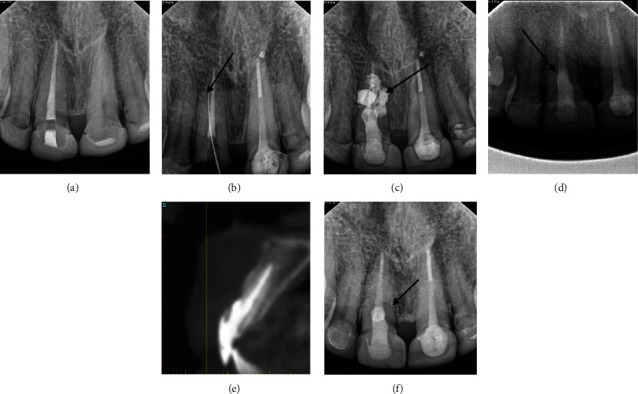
(a) Radiograph after root canal retreatment. (b) Radiograph showing root perforation after postspace preparation. (c) Calcium hydroxide placement. (d) MTA placement. (e) Cone beam CT scan 18 months after MTA (prior to our intervention). (f) Follow-up after 5 years of Geristore® placement.
